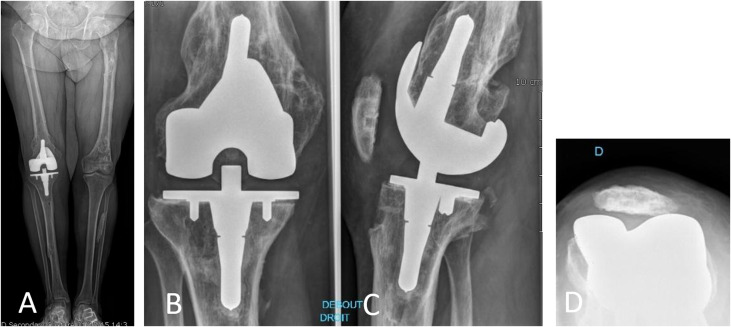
Post-operative plain radiographs: (A) standing both lower limbs, (B) AP, right knee, (C) lateral view, right knee, (D) merchant view, right knee.
